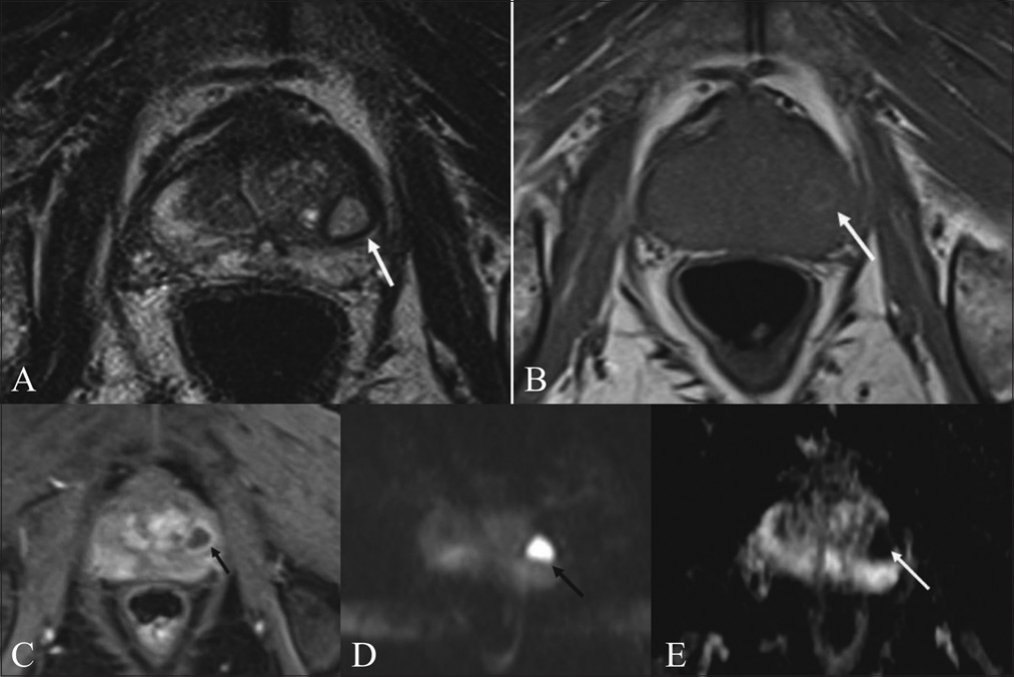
Prostatic abscess – case 2. Axial T2W MRI of the prostate (A) shows high signal (arrow) in the central gland, in the left midzone. Axial T1W image (B) shows mixed signal intensity with peripheral hyperintensity (arrow). Axial, contrast-enhanced T1W image (C) shows a peripherally enhancing abscess (arrow). DWI (b=800) (D) shows restriction of diffusion (arrow) in the lesion. The corresponding ADC map (E) shows low signal (arrow)
